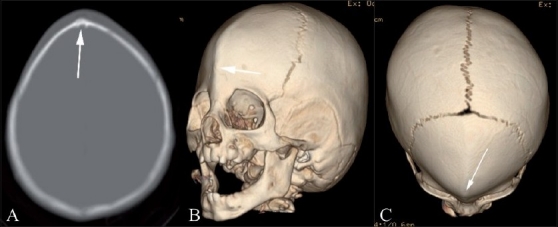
Trigonocephaly. Axial CT scan (A), anterolateral (B) and superior (C) 3DCT volume rendered images show trigonocephaly from premature / abnormal fusion of the metopic suture (arrow). Note marked flattening of the frontal bones
